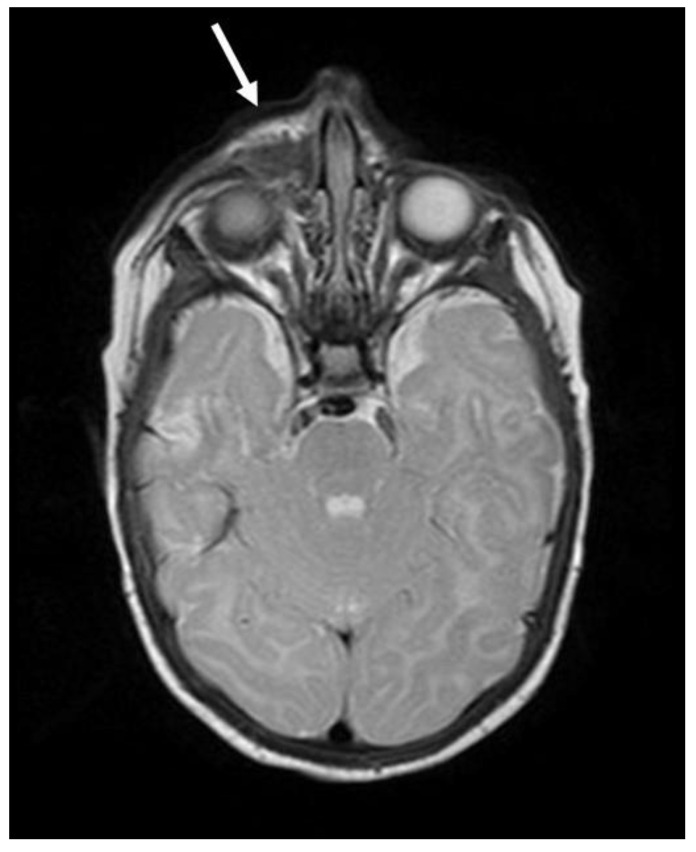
Patient 1: an axial T2-weighted MR scan shows recurrence of MNTI in the postoperative area.
